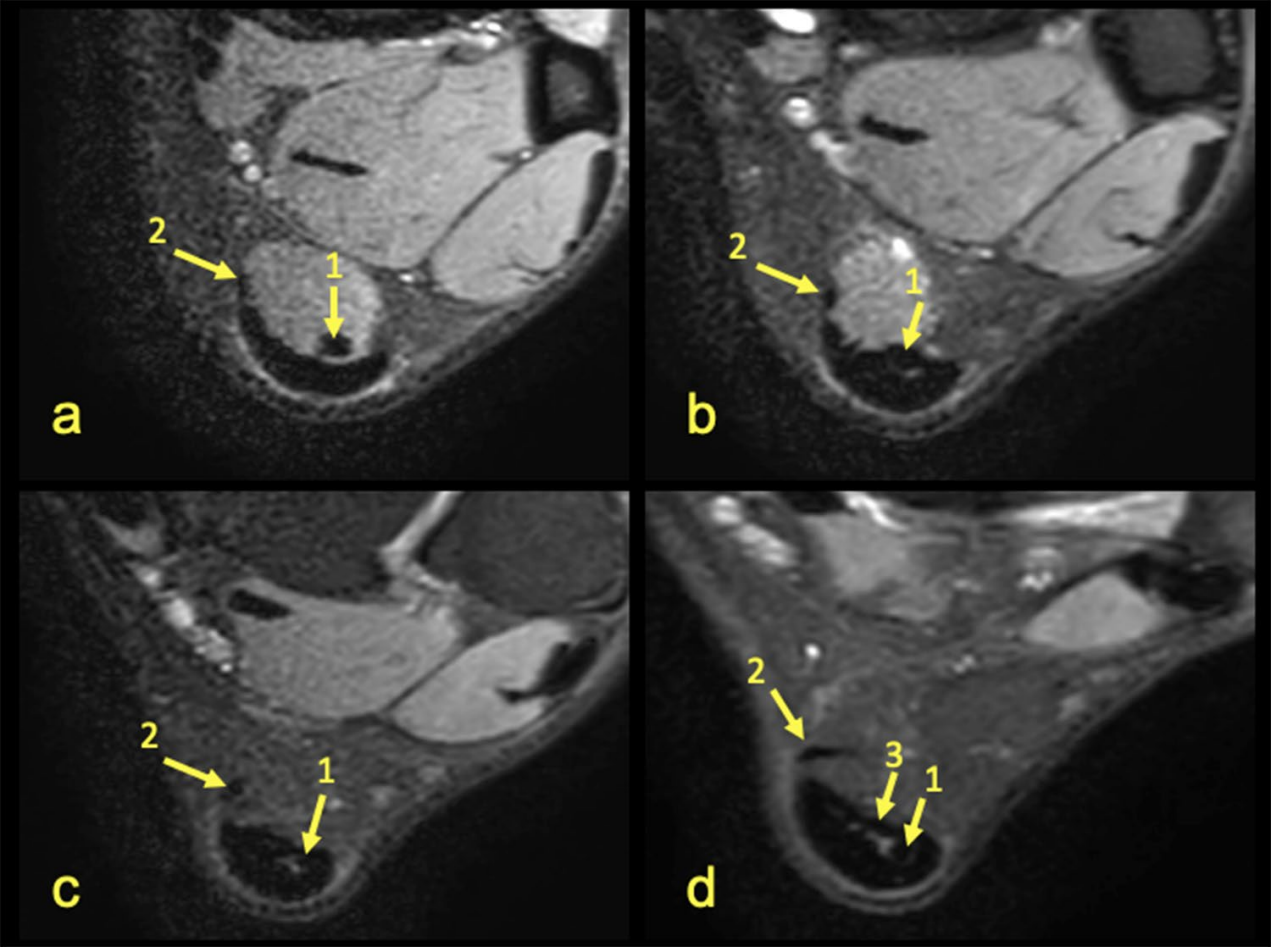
MRI left ankle, PD FS at the level of the talus; (a)—the highest section, (d)—the lowest section. A 48-year-old male presented with the anterior ankle pain. 1—S-Sol, 2—the plantaris tendon (type 5), 3—S-LGC.
